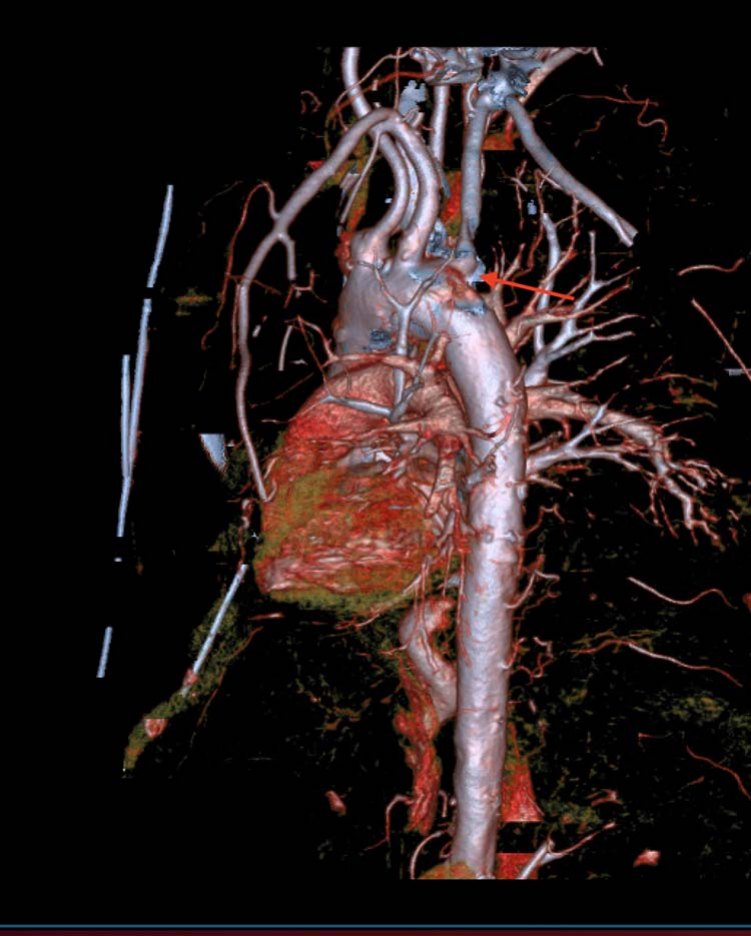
CT thoracic showing the arch of the aorta with the take-off of the right subclavian artery (red arrow) distal to the left subclavian artery.
